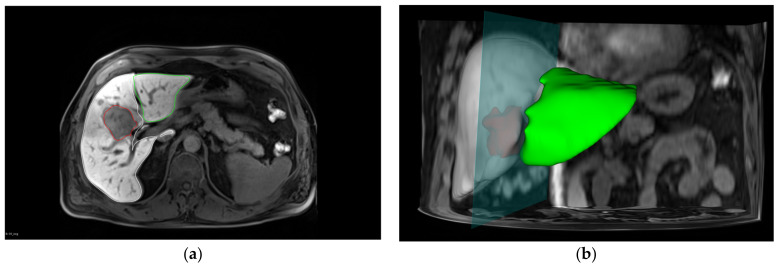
Virtual operation planning. (a) Axial MRI scan of the liver in the hepatobiliary phase using a VIBE sequence. The liver contour is outlined to facilitate liver segmentation. The tumor region is represented in red, the resected liver lobe in white, and the remaining liver lobe in green. (b) A 3D visualization of the virtual operation planning, providing a comprehensive spatial understanding of the liver structure pre- and post-operation, as well as the location and extent of the tumor and the resected liver lobe.
